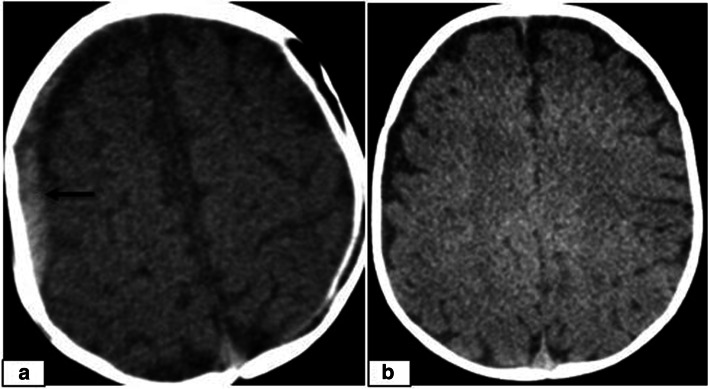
A 9-month-old female girl presenting with nausea and vomiting 25 days after undergoing allogeneic HSCT for SCID. a Axial CT image showing acute hyperdense right frontoparietal SDH (arrow). b Follow-up axial CT image 1 month after the onset of acute SDH showing complete resolution
